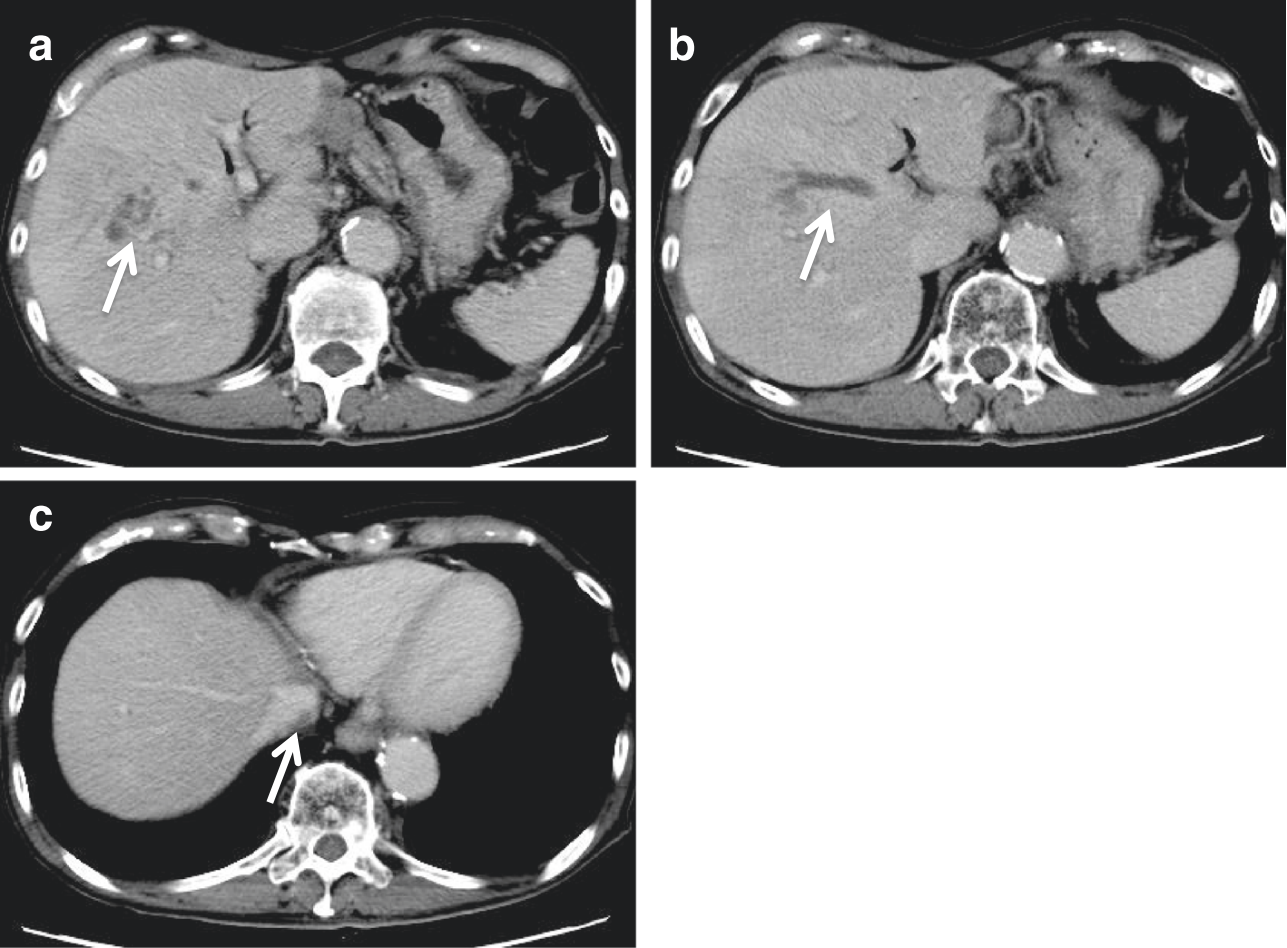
Follow-up computed tomography on hospital day 8. a The abscess cavity was reduced slightly (*arrow*). b We detected a thrombus in the middle hepatic vein (*arrow*). c The thrombus extended to the inferior vena cava (*arrow*)
